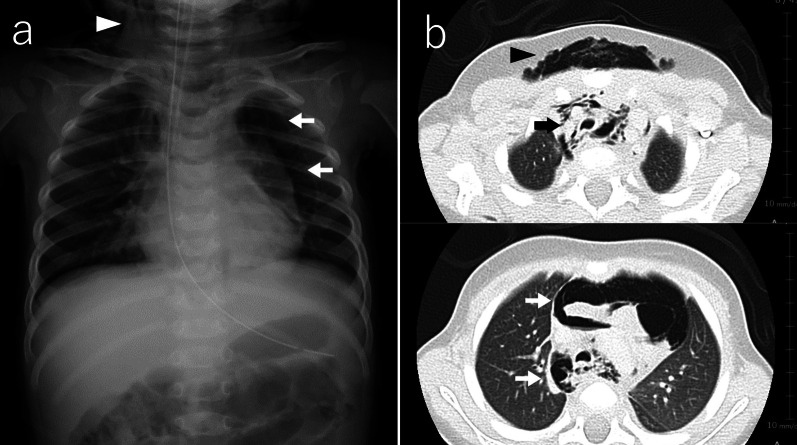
Chest X-ray (a) and a CT scan (b) on admission. A chest X-ray and a CT scan revealed extensive subcutaneous emphysema extending in the neck (arrow) and the pneumomediastinum (arrowhead)
